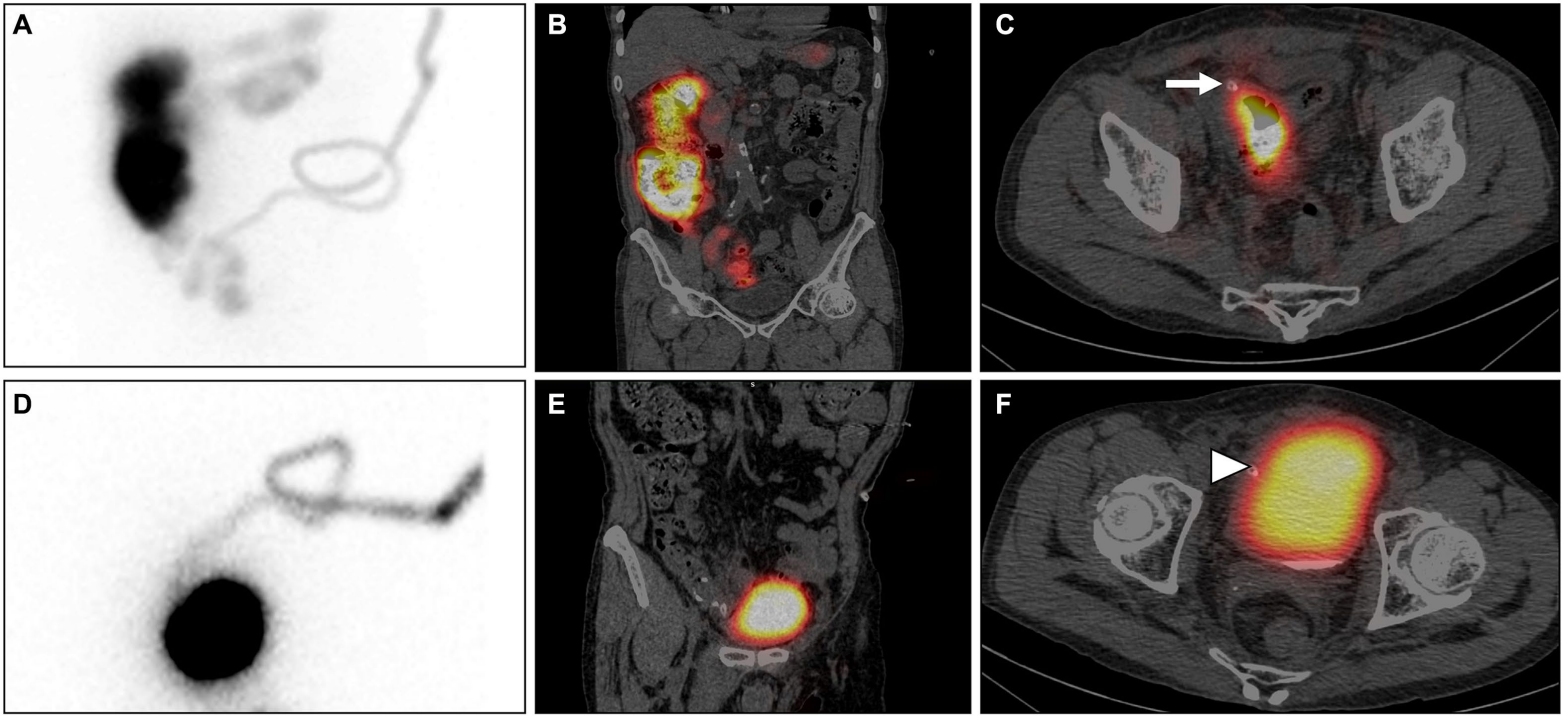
99mTc-NaTcO4 SPECT/CT: The Maximum Intensity Projection (MIP) image (A) and coronal SPECT/CT (B) demonstrate radiotracer uptake in the intestines, while the axial SPECT/CT (C) indicates the site of perforation at the terminal ileum (arrow). After conservative treatment, re-examination with SPECT/CT shows radiotracer uptake in the bladder and no radiotracer uptake in the intestines on the MIP (D) and coronal SPECT/CT (E). The axial SPECT/CT (F) confirms the presence of bladder perforation (arrowhead).
